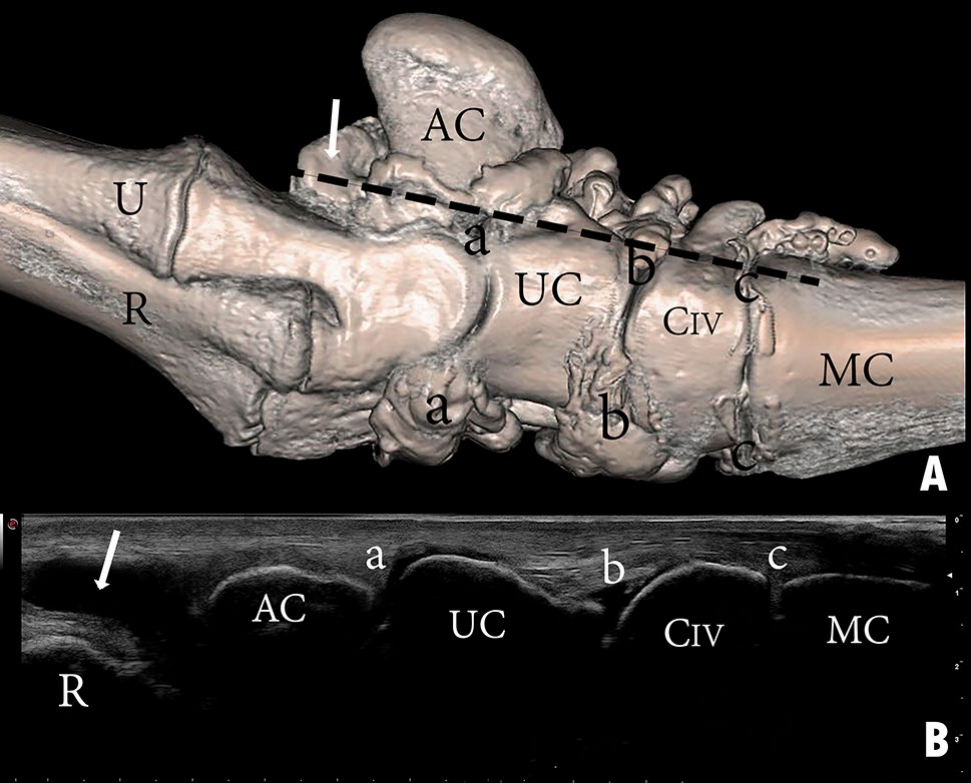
Lateral view of a left carpal joint showing (A) three-dimensional reconstruction of the carpal joint (the black dotted line indicates the lateral placement of the ultrasound probe); (B) longitudinal composed ultrasound image of the carpal joint. R, the distal end of the radius; U, the distal end of the ulna; AC, accessory carpal bone; UC, ulna carpal bone; CIV, carpal bone IV; MC, metacarpal bone; a, radiocarpal joint cavity; b, intercarpal joint cavity; c, carpometacarpal joint cavity. The white arrow indicates the lateropalmar pouch of the radiocarpal joint.
